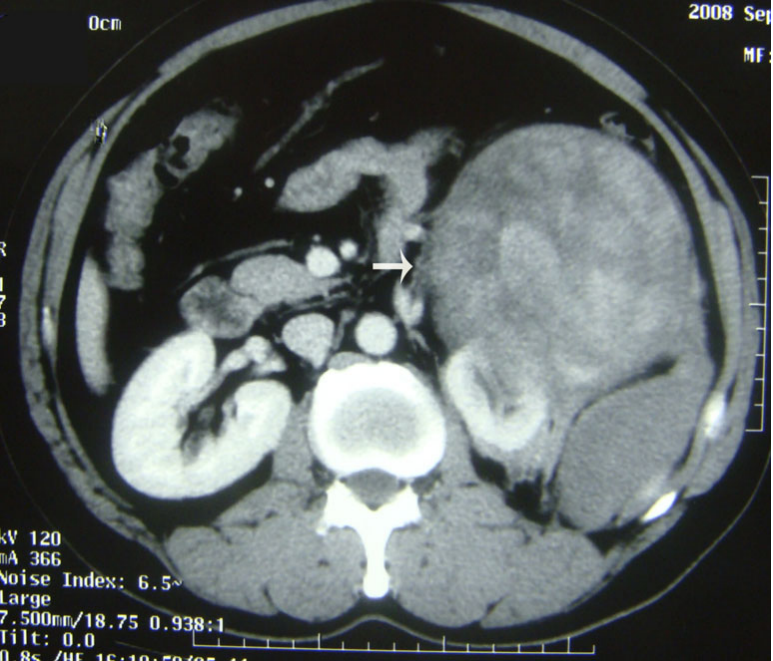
Computed tomography (CT) showing a giant mass located in the retroperitoneal space with invasion to the left kidney.
